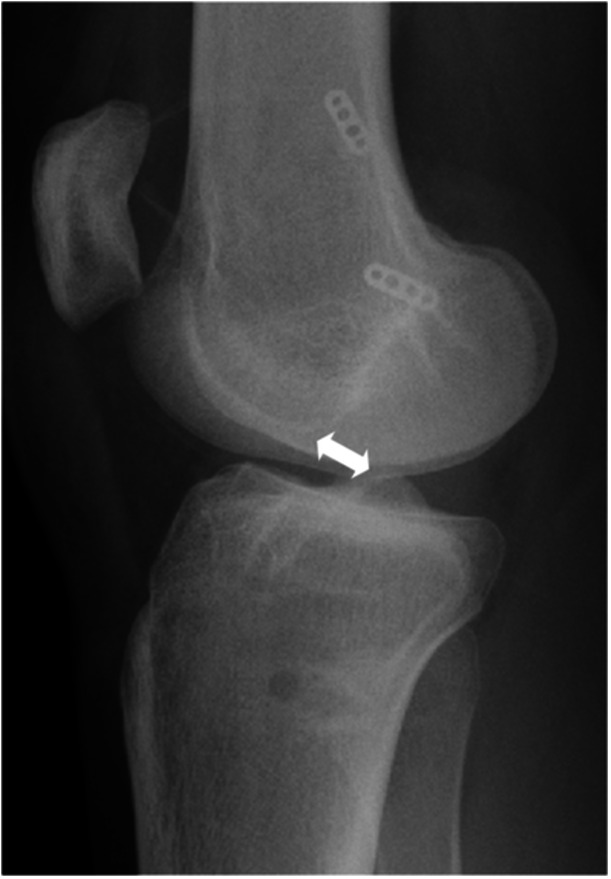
Radiographic measurements (space for the anterior cruciate ligament). Measurement of space for the anterior cruciate ligament. The distance between the tip of the tibial eminence and the most inferior portion of the Blumensaat line.
